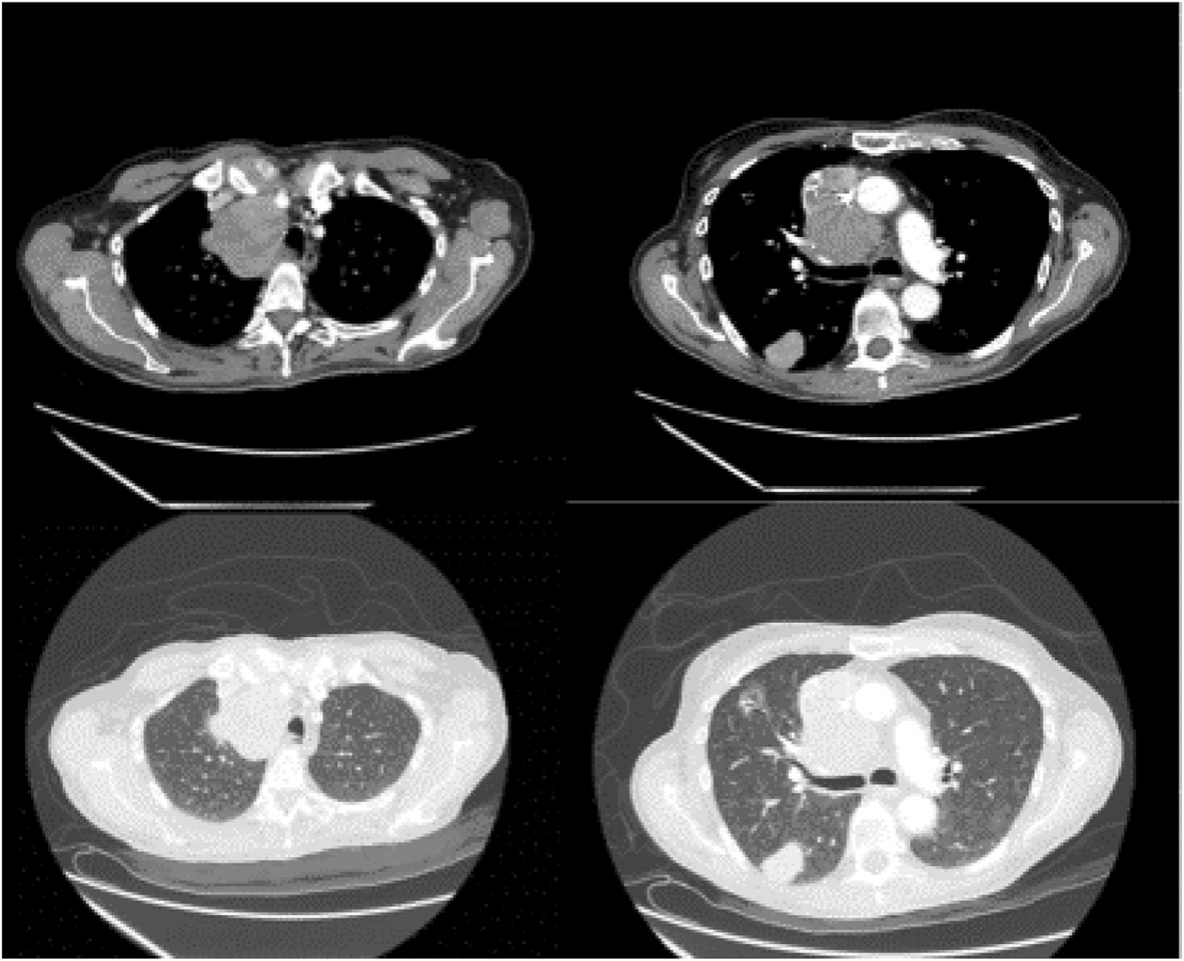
Chest enhanced computed tomography (CT) showed a solid lesion in the apical segment of right lower lobe with multiple confluent mediastinal adenopathies and right paratracheal lymphadenopathy
